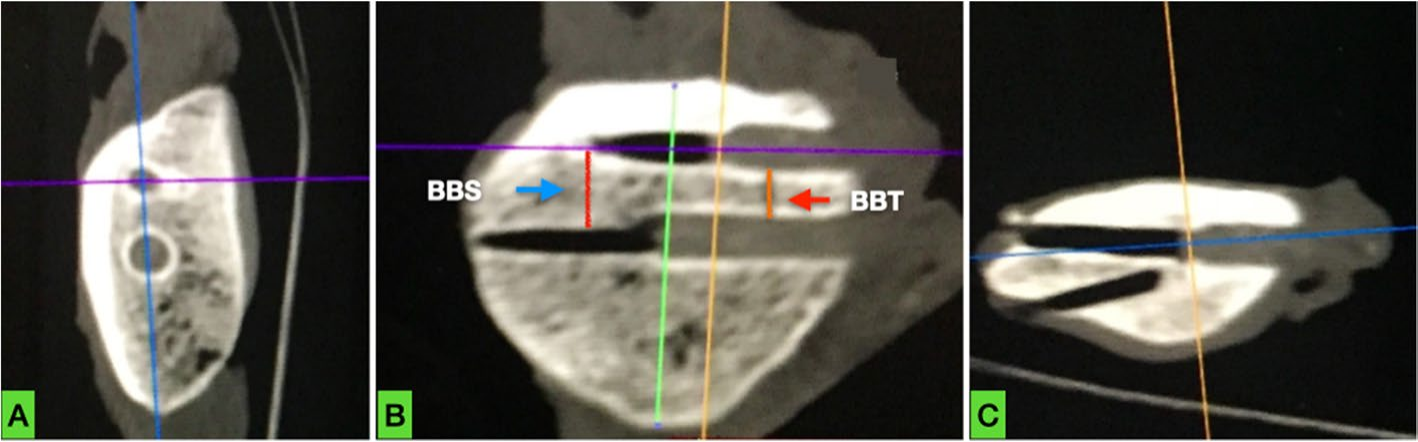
Sagittal, frontal and axial CT section of patella #10 postoperatively. Tunnel placement in a and b appears adequate. However, in c, two attempts made by the surgeon for proximal tunnel placement are revealed. The surgeon was probably unable to create a correct tunnel between previous attempted drillings. Blue arrow in b points at the postoperative bone bridge (red line) between transosseous sutures (BBS). Red arrow in b points at the postoperative bone bridge (orange line) between tunnels (BBT)
